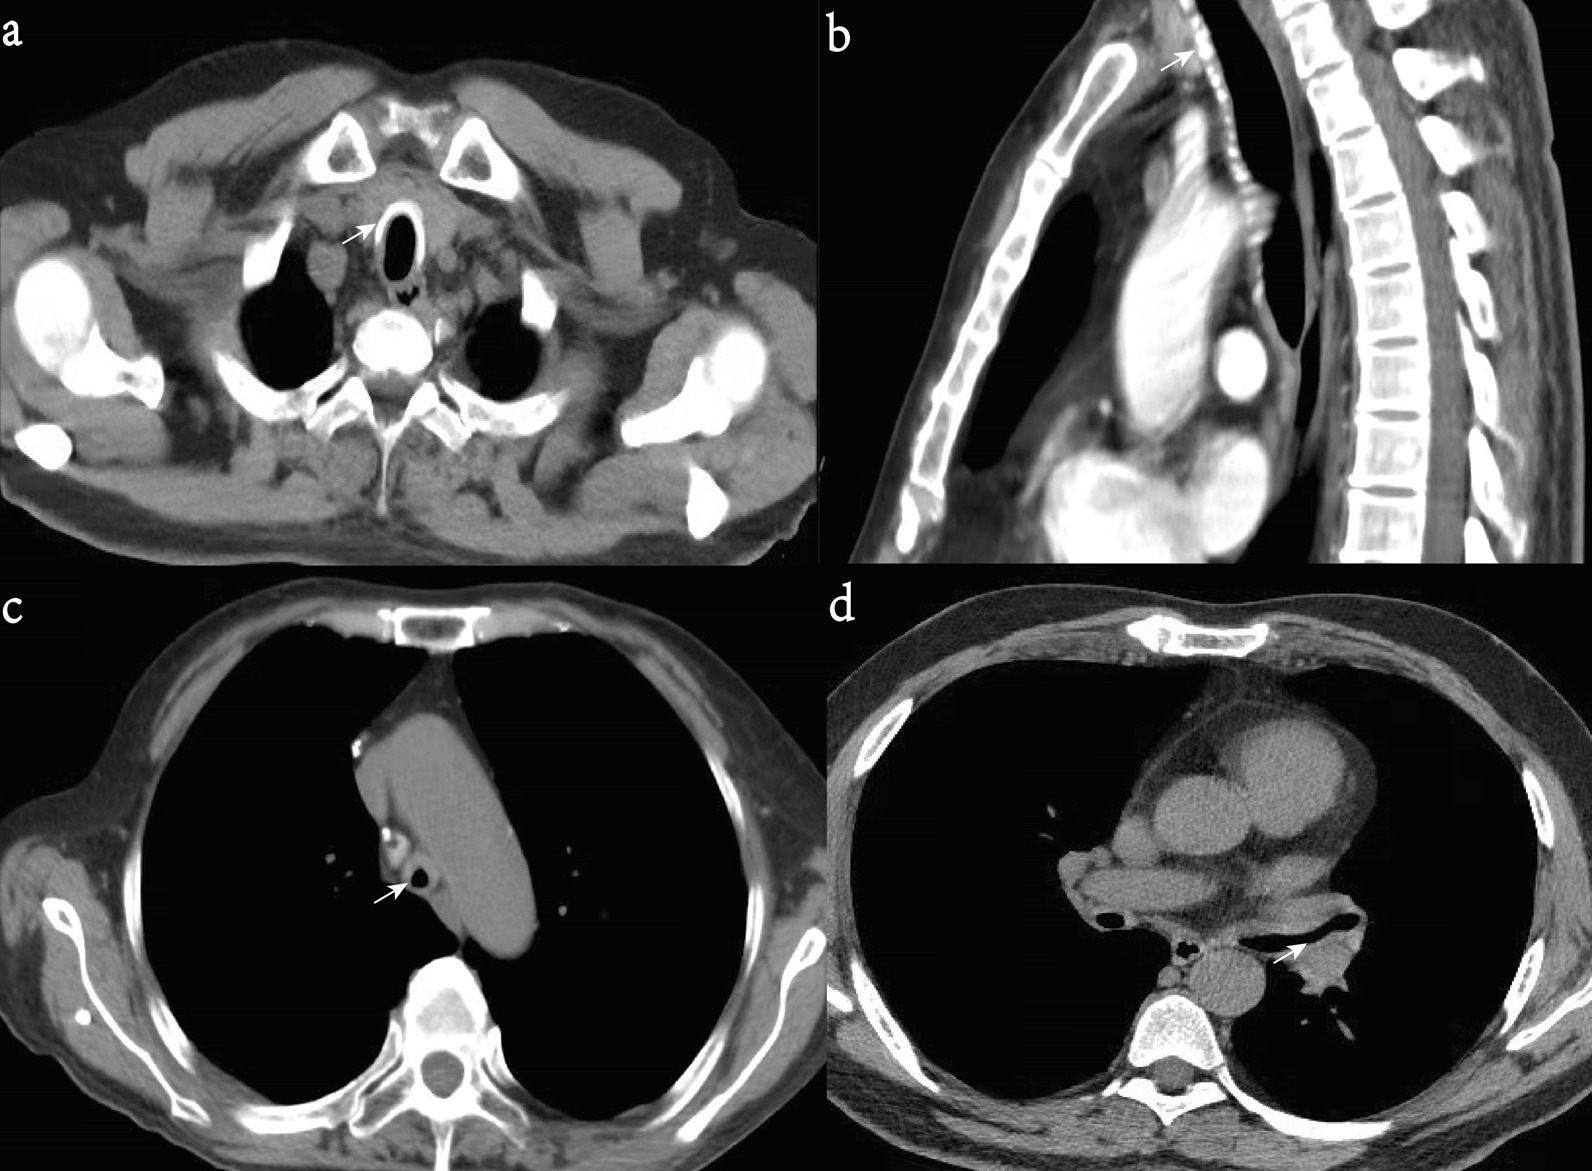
a Axial CT slices showing tracheal wall thickening contains calcific deposits (arrow). b Coronal CT reconstruction showing diffuse thickening of the anterior wall of the trachea, with calcifications (arrow) in a 47-year-old man with relapsing polychondritis. Axial CT slices showing airway stenosis (arrow) of the trachea (c) and left main bronchus (d) in a 56-year-old man with relapsing polychondritis
